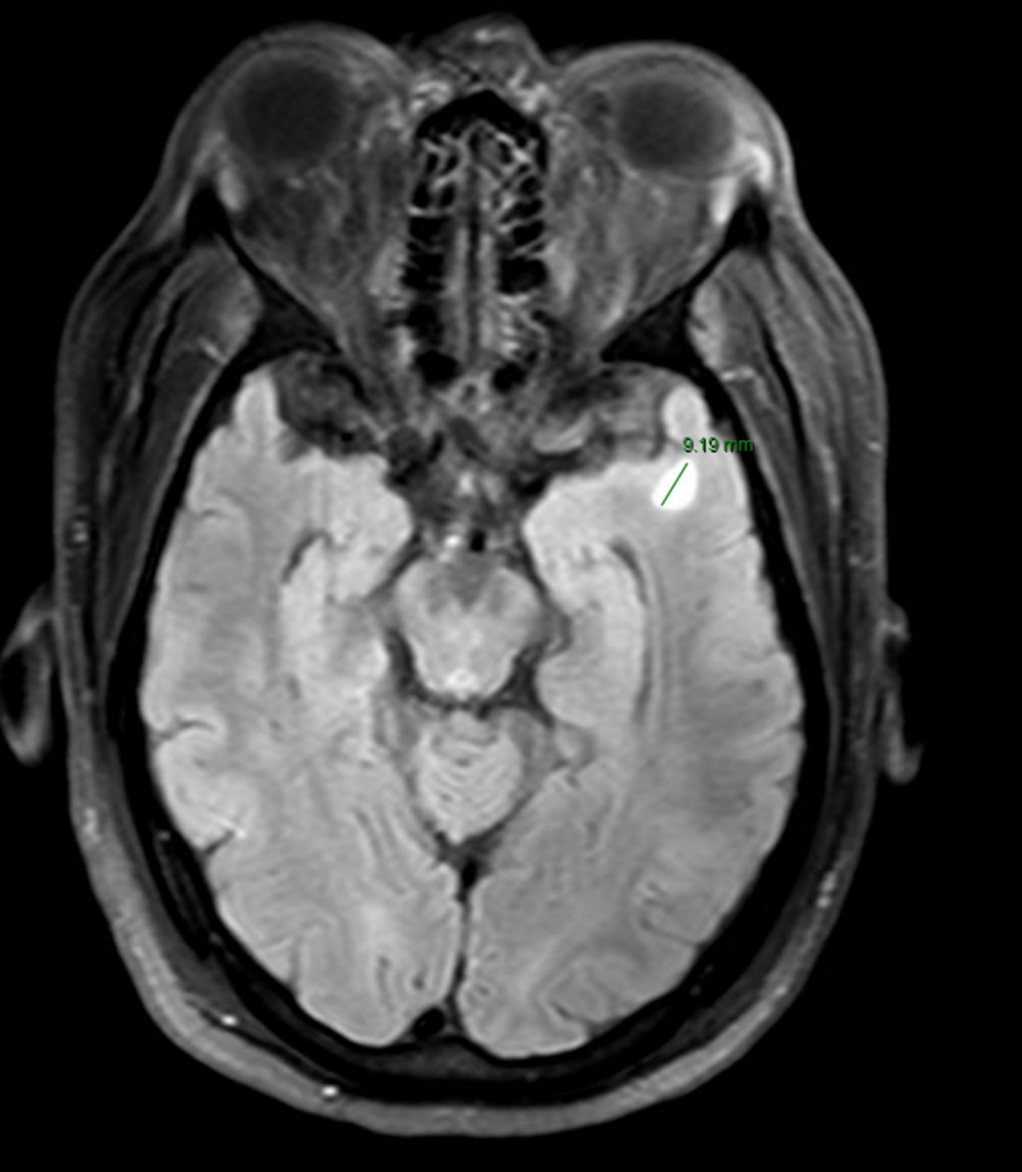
Magnetic resonance imaging (MRI) of the brain. The arrow in the scan depicts a non-enhancing lesion in the left temporal lobe of the brain, which reflects either a low-grade primary brain malignancy or a metastatic disease.
